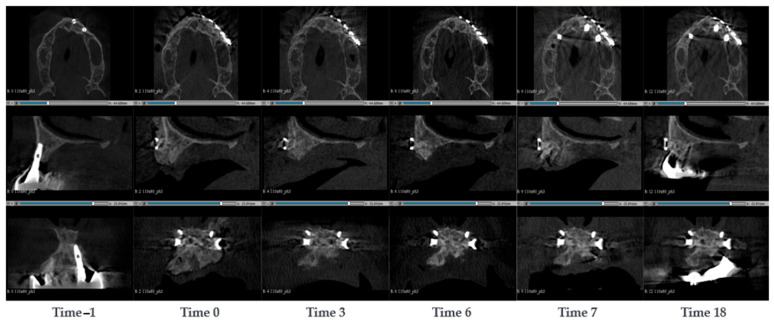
CBCT images of a 54-year-old woman with bone resorption around a prosthetic arch in the maxilla. The defect was repaired by a fencing procedure, filled with SmartBone^®^ microchips. The images show the progression of bone healing over time, from pre-surgery (Time −1) to 18 months post-surgery (Time 18).
